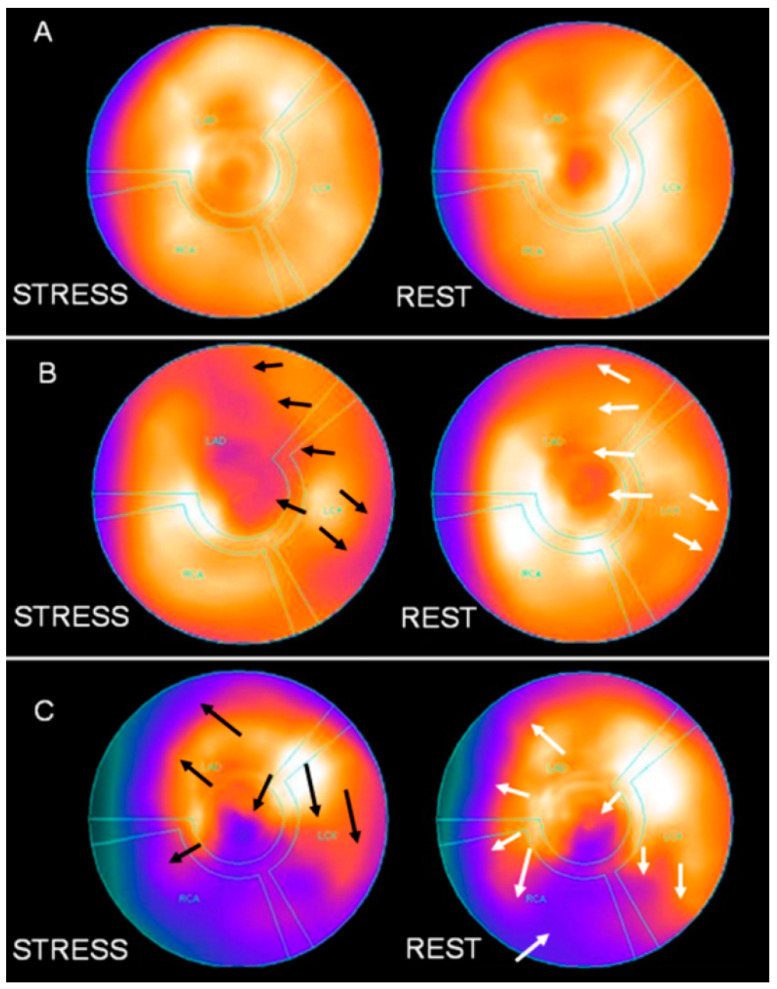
A summed stress score (SSS) > 3 indicates an abnormal MPI-SPECT, considered with myocardial ischemia or a myocardial infarct. Additionally, according to the score, myocardial ischemia may be graded as mild (4 ≤ SSS < 9), moderate (9 ≤ SSS < 14), or severe (SSS > 14). Summed rest score (SRS) indicates defects at rest, defects attributed to a scar, or defects attributed to a hibernating myocardium. Finally, the difference between SSS and SRS indicates the reversibility of ischemia induced by stress (exercise or pharmacologic stress such as in our cases). Here, we present three different MPI-SPECTs. Black arrows show the defects in stress and white arrows indicate the reversibility (complete or partial) in MPI-SPECT. (A) The MPI-SPECT did not reveal any defects and it was diagnosed as normal. (B) The MPI-SPECT revealed mild ischemia involving the cardiac apex of the anterior and basal lateral cardiac wall. The SSS and SRS were 7 and 0, respectively. The SDS was 7, indicating a reversible myocardial ischemia. (C) The MPI-SPECT revealed severe ischemia, involving the cardiac apex, lateral apical, middle and basal infero-lateral, the inferior and the middle and basal infra-septal cardiac wall (SSS—23, SRS—10 and SDS—13), indicating a moderately reversible myocardial ischemia.
